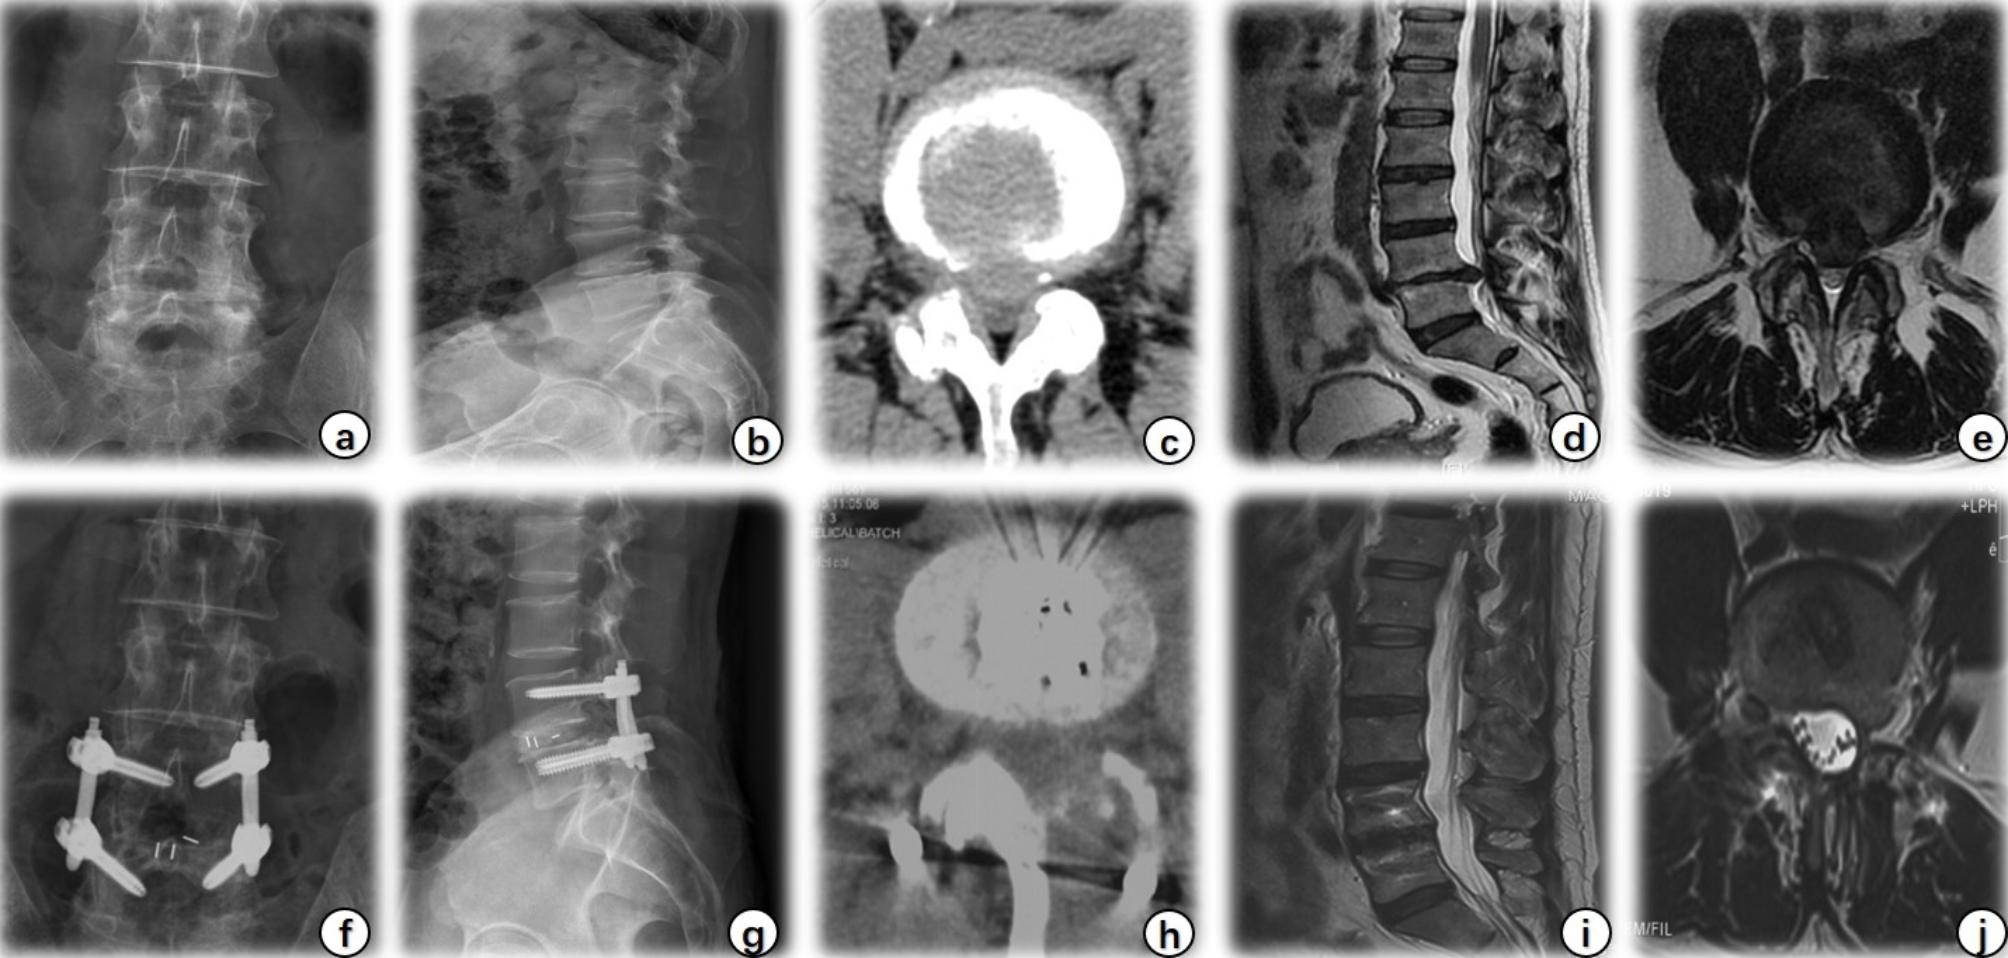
A typical BE-LIF case. A 57-year-old man presented with recurrent lower back pain radiating to the left lower limb with intermittent claudication for six years, which worsened and failed to respond to conservative treatment for six months. Diagnosis: lumbar disc herniation with spinal stenosis. A, B: Preoperative anteroposterior and lateral X-rays of the lumbar spine; c, d, e: Preoperative axial CT, sagittal and axial MRI showed L4/5 lumbar disc herniation with spinal canal stenosis; f, g: Postoperative anteroposterior and lateral X-rays of the lumbar spine; h: Axial CT after BE-LIF showed sufficient spinal canal decompression and local bone defect; i, j: Postoperative sagittal and axial MRI indicated adequate decompression
